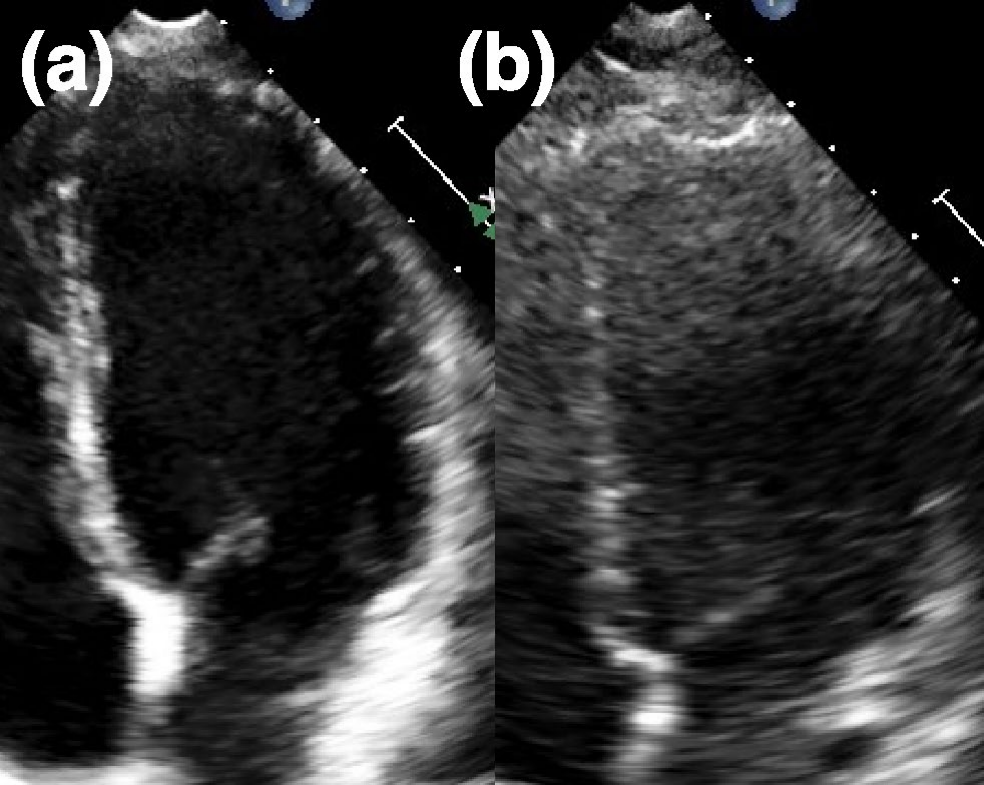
Demonstration of contrast-to-noise (CNR) differences for echocardiogram scans from clinical test subjects. (a) High CNR (> 8 dB), where there is little signal within the LV and strong tissue signal in the myocardium, composing the LV. (b) Low CNR (< 2 dB), where there is high, incoherent speckle noise within the LV and weak tissue signal along with signal loss within the myocardium composing the LV. These images were taken from two female subjects, one aged 21 years old (y/o) and the other aged 24 y/o.
